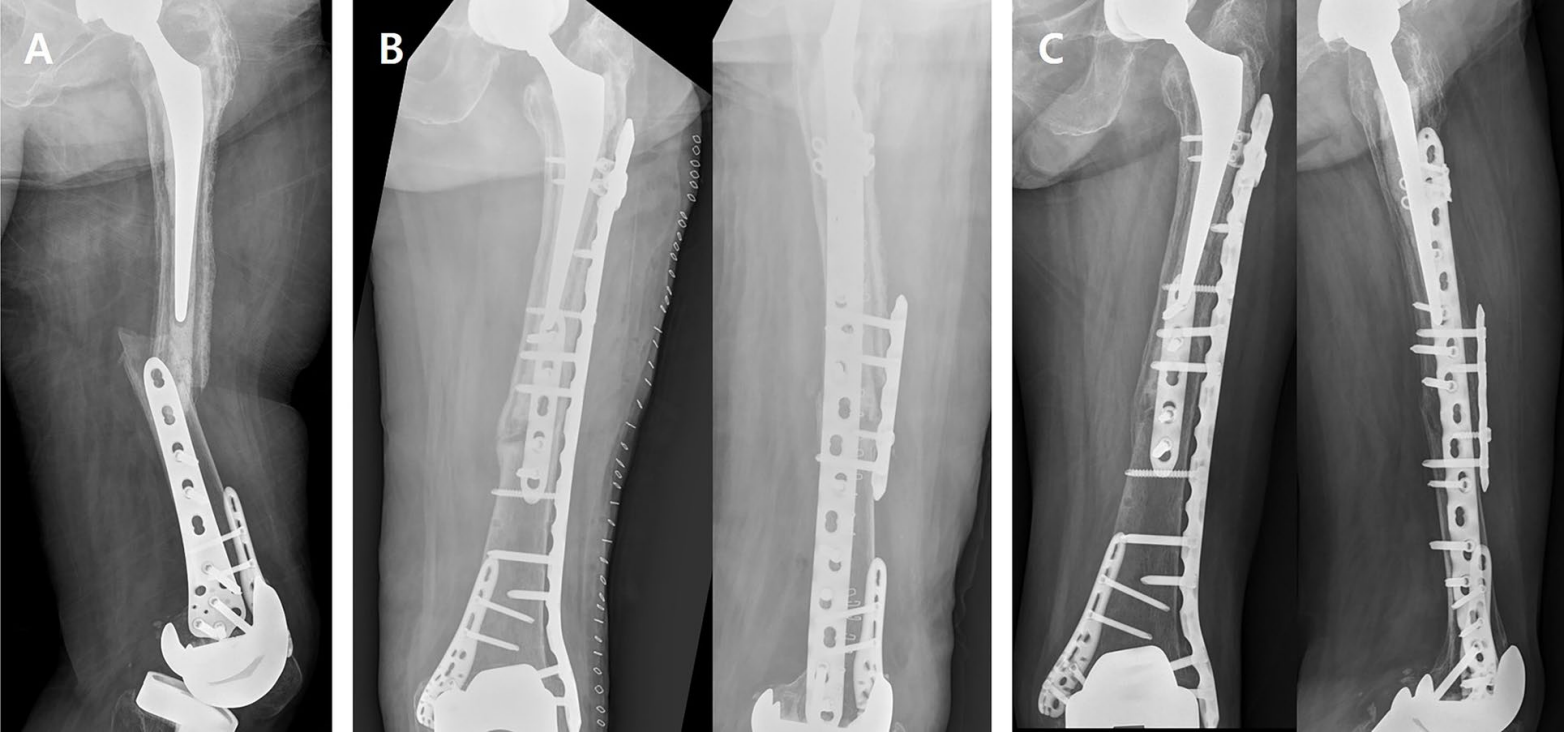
A A preoperative plain radiograph of a 68-year-old female who sustained a periprosthetic femoral shaft fracture following THA, representing a Vancouver type C. A 4.5 mm short locking plate fixation was initially used to reduce the fracture. The patient had already undergone dual-locking plate fixation for an extremely distal femoral periprosthetic fracture after TKA, 3 years ago. B Pre-existing anatomical short locking plate (DF plate) was removed. The patient was treated with a 4.5-mm LCP long broad-curved plate overlapping the zone of the stem at least 6 cm. Intraoperative plate bending was performed using a large bender to fit the proximal and distal femoral geometry. Supplementary fixation using a LAP was performed in the overlapped zone. C At 5 months postoperatively, a solid bone union was acquired. THA, total hip arthroplasty; TKA total knee arthroplasty; DF, distal femur; LCP, locking compression plate; LAP, locking attachment plate
